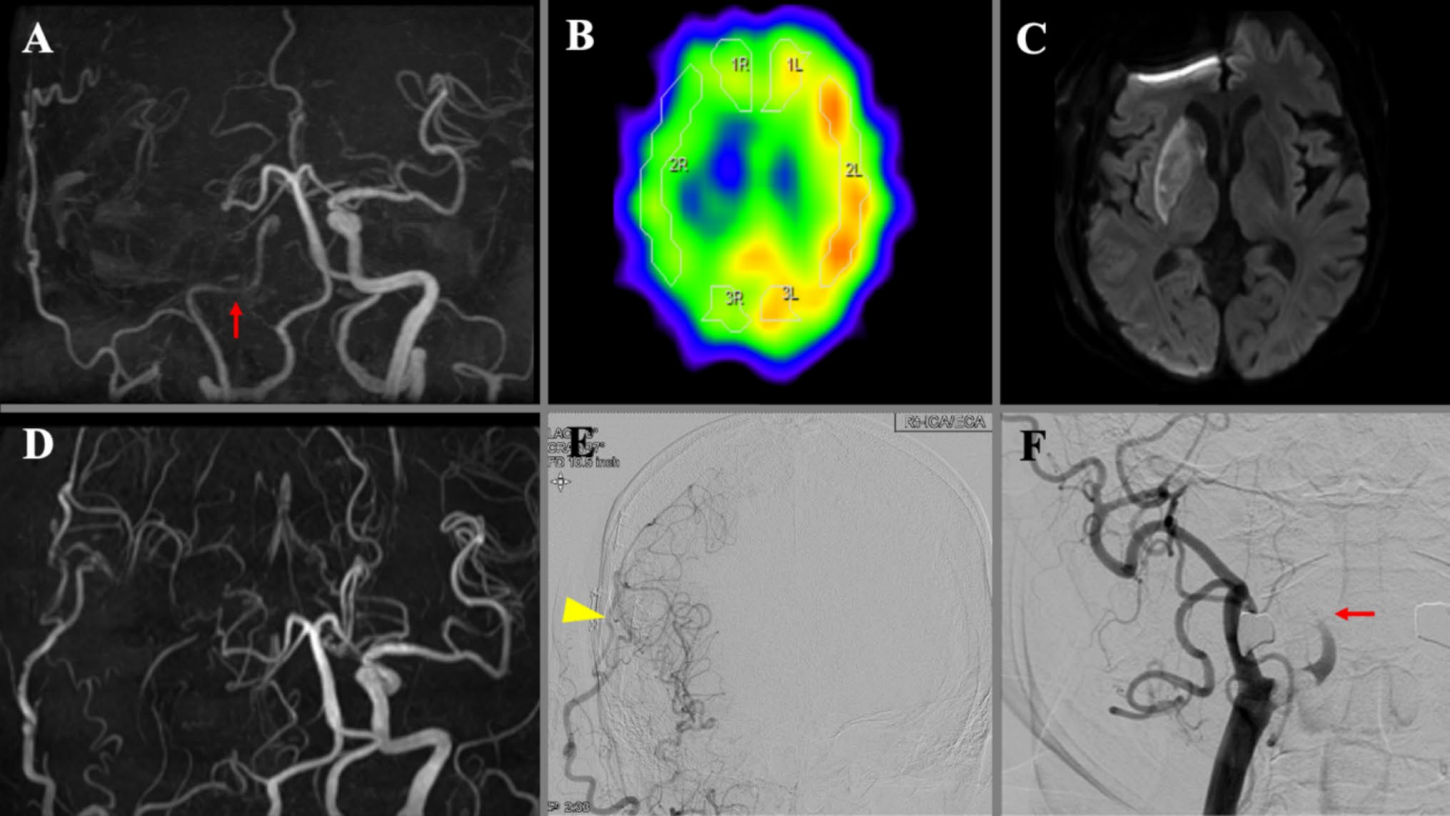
A Preoperative MRA shows a diminished flow signal in the right ICA due to severe stenosis at the petrous segment (red arrow). B Preoperative SPECT demonstrates markedly reduced cerebral blood flow and cerebrovascular reactivity in the right anterior circulation. C, D Diffusion-weighted MRI on postoperative day 3 reveals a new infarction in the right basal ganglia (C), despite good bypass patency confirmed by MRA (D). E, F Postoperative digital subtraction angiography from right intracranial (E) and carotid (F) injections shows that the STA–MCA bypass (arrowhead) supplies the entire peripheral MCA territory. Anterograde flow from the right ICA is interrupted just distal to the carotid bifurcation
